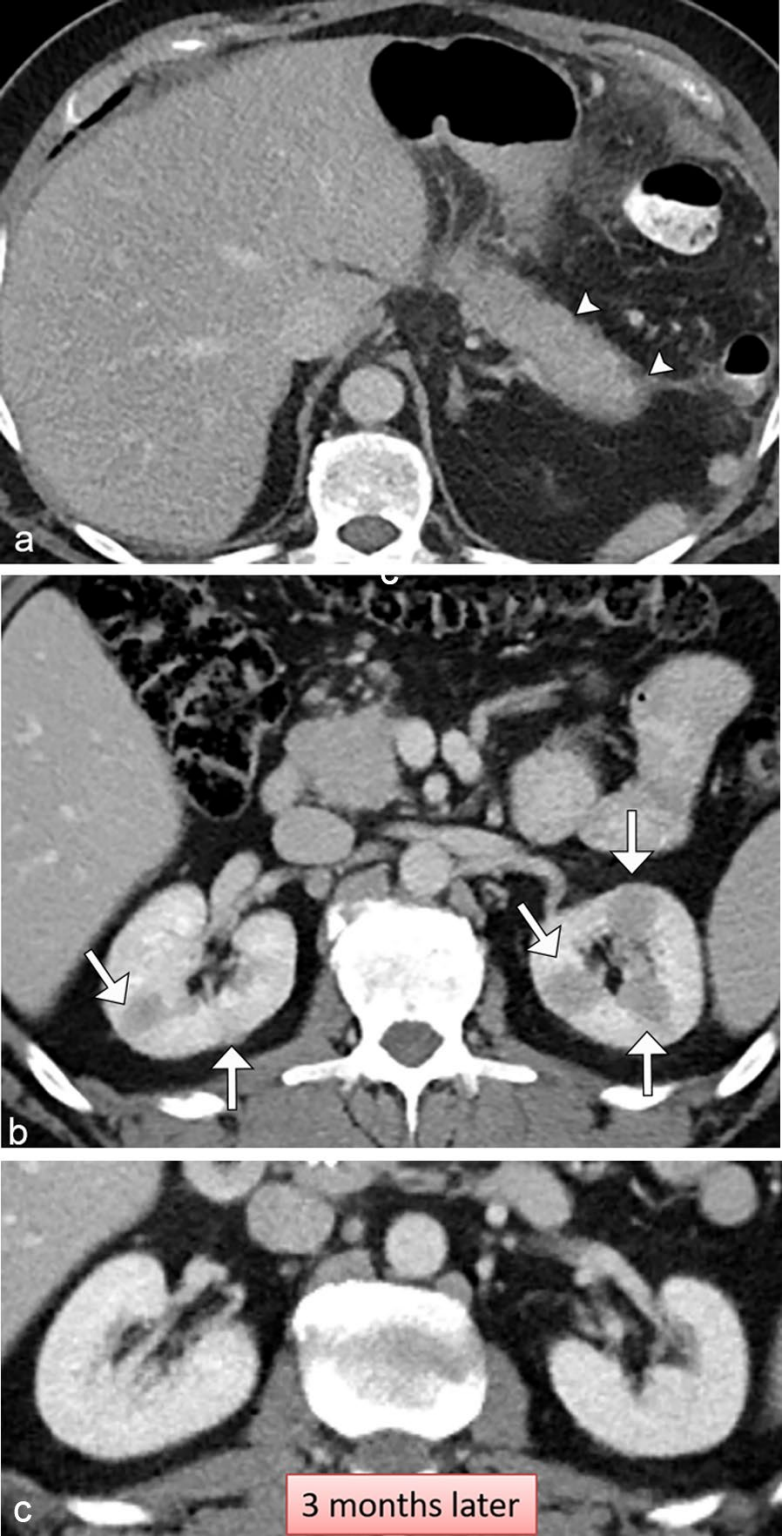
IGG4-related renal disease*. Axial contrast CT images demonstrate (a) a sausage-shaped pancreas with loss of normal pancreatic lobulations and presence of a hypoattenuating surrounding halo/capsule (arrowheads) consistent with IGG4-related autoimmune pancreatitis. (b) Bilateral hypoattenuating renal masses (arrows) were present compatible with IGG4-related renal disease in light of the pancreatic findings, mimicking the multiple masses of lymphoma. Axial contrast CT 3 months later (c) post-treatment with steroids demonstrates resolution of the renal masses confirming the initial diagnosis
